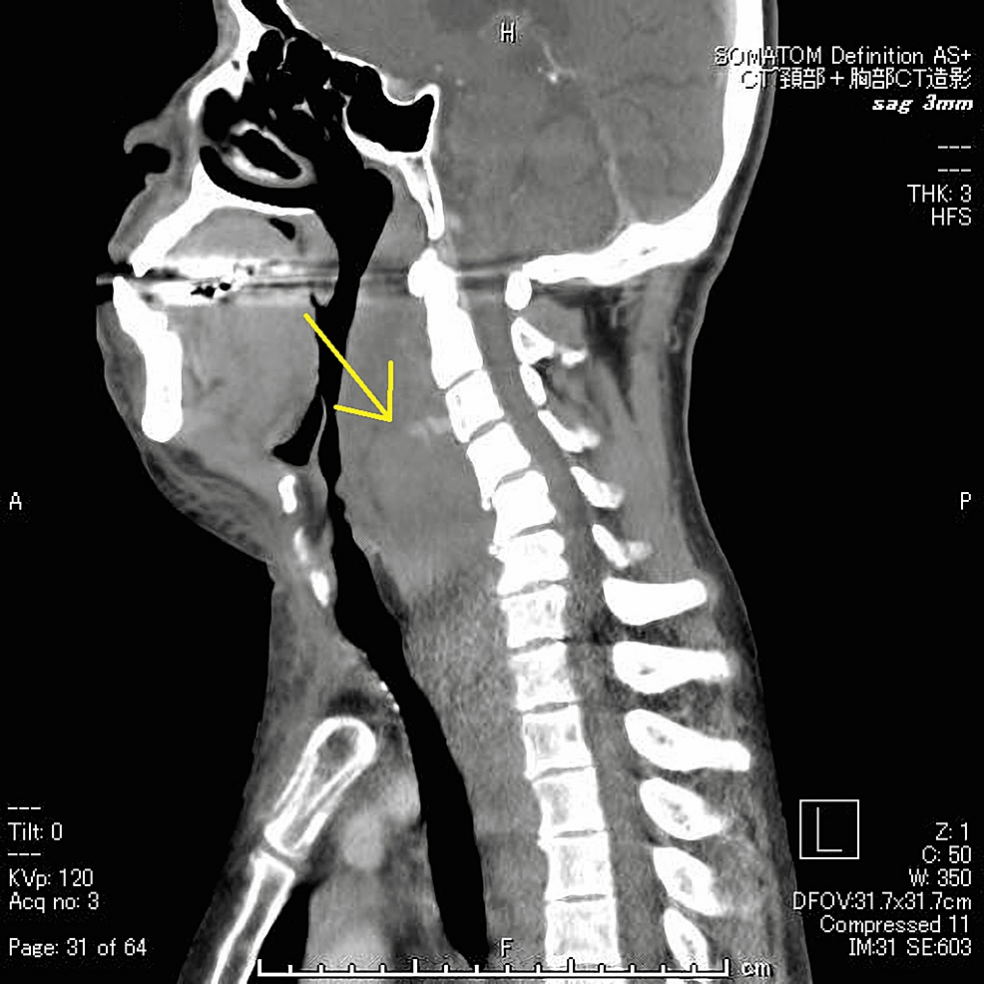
Cervical contrast CT at initial examination (sagittal) The arrow points to the high-density area, which was considered a hematoma.
